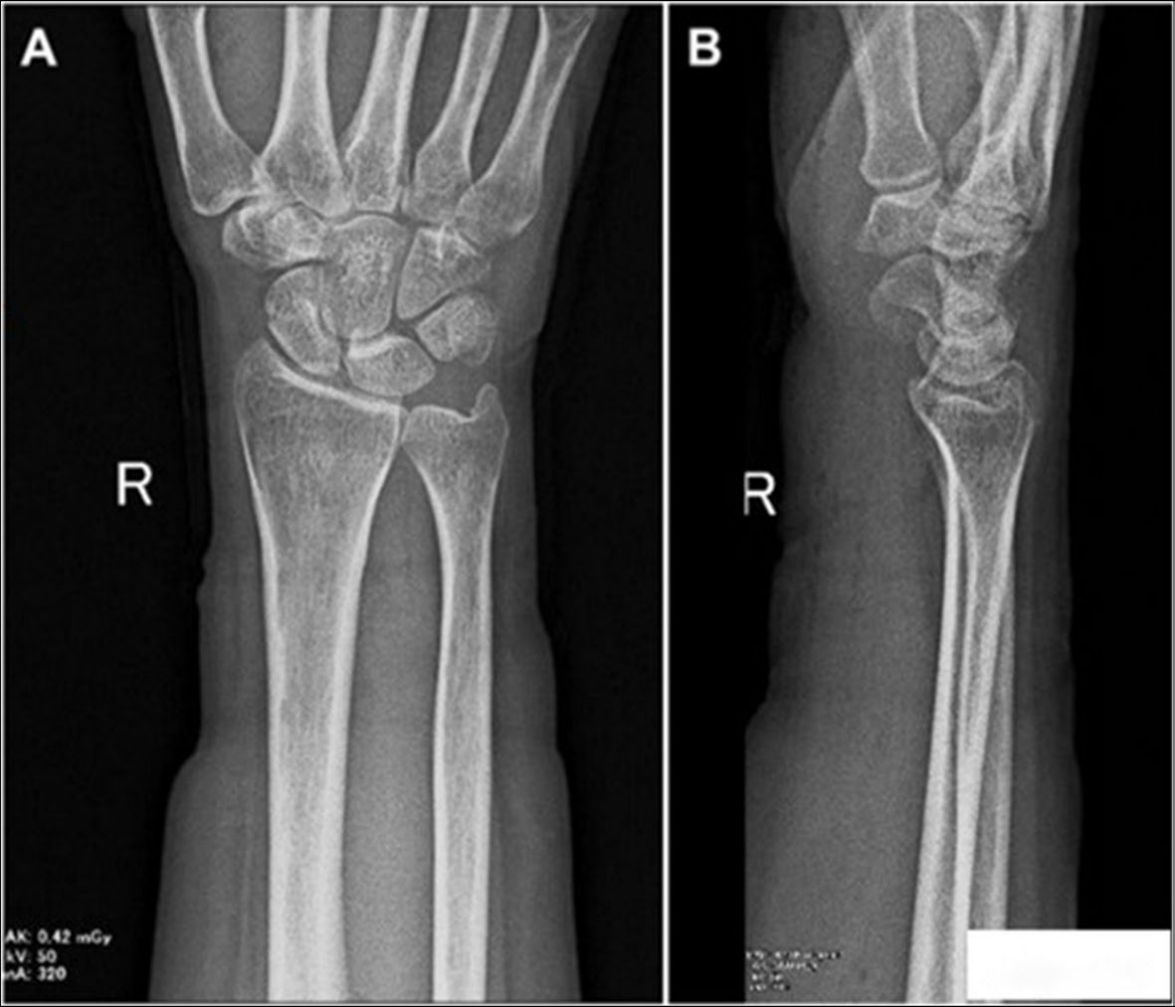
Postoperative plain radiograph following removal of the calcification. A Postero-anterior view. B Lateral view
